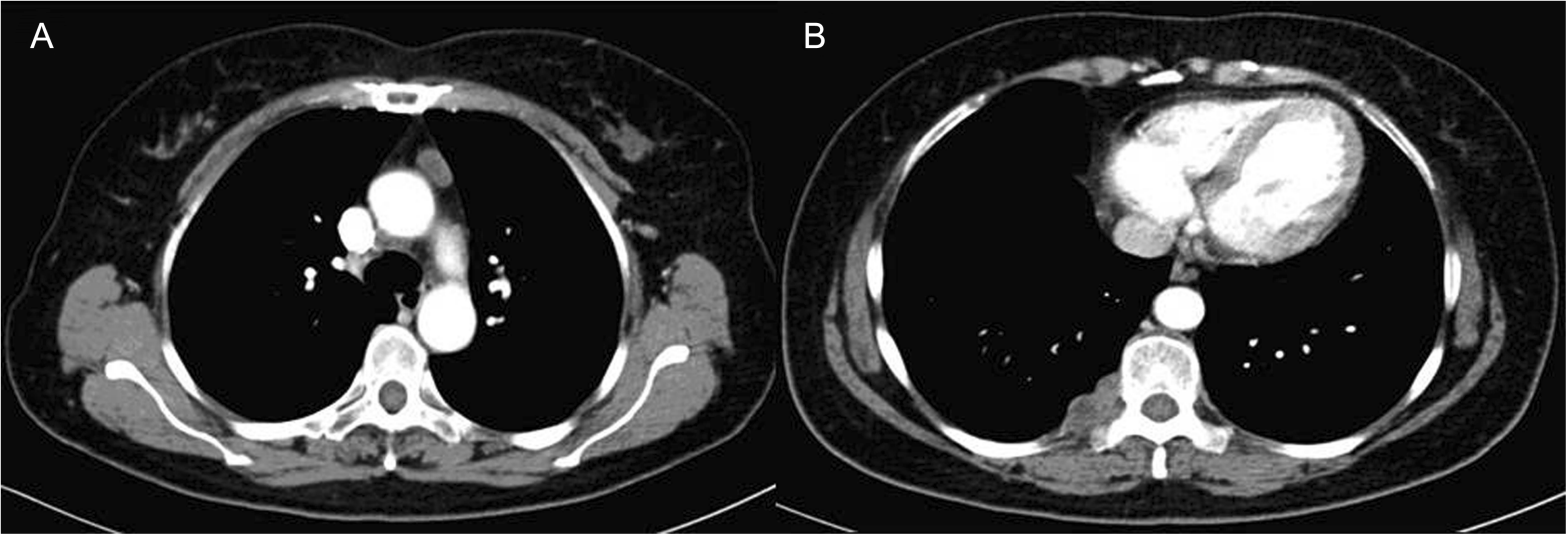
CT scan of the chest revealed that two tumors located at the left-sided anterior mediastinum (a) and paravertebral right-sided lower thorax (b), respectively
